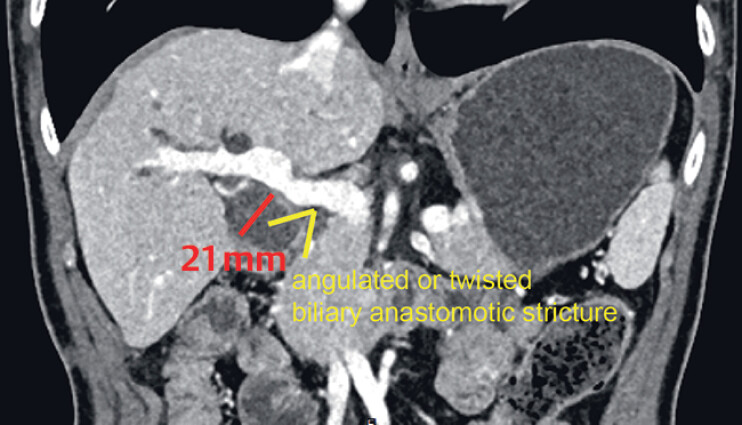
Contrast-enhanced abdominal computed tomography (coronal plane) revealed an angulated or twisted biliary anastomotic stricture, with a dilated common bile duct measuring 21 mm.
